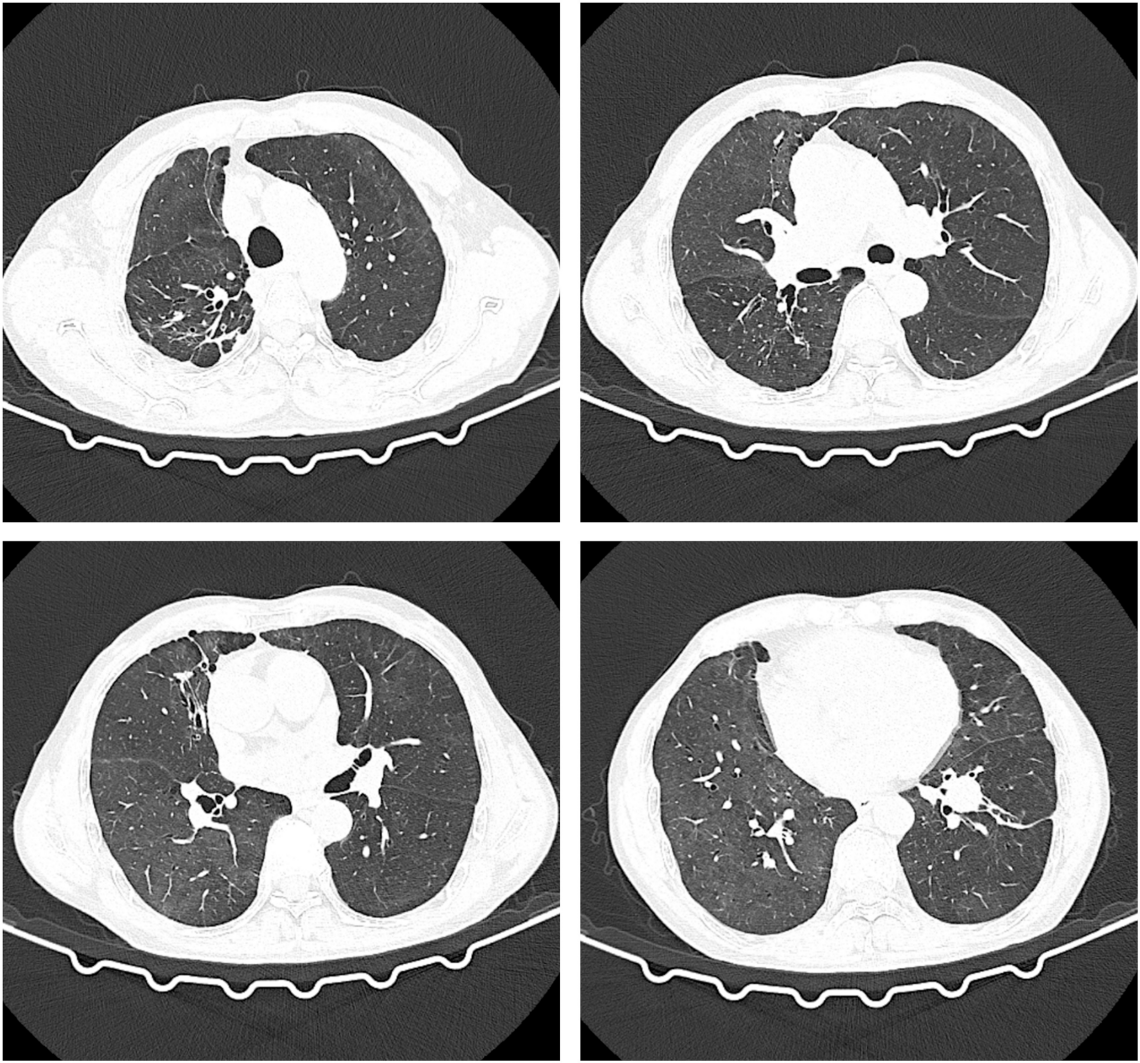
The Chest CT on August 22nd.
